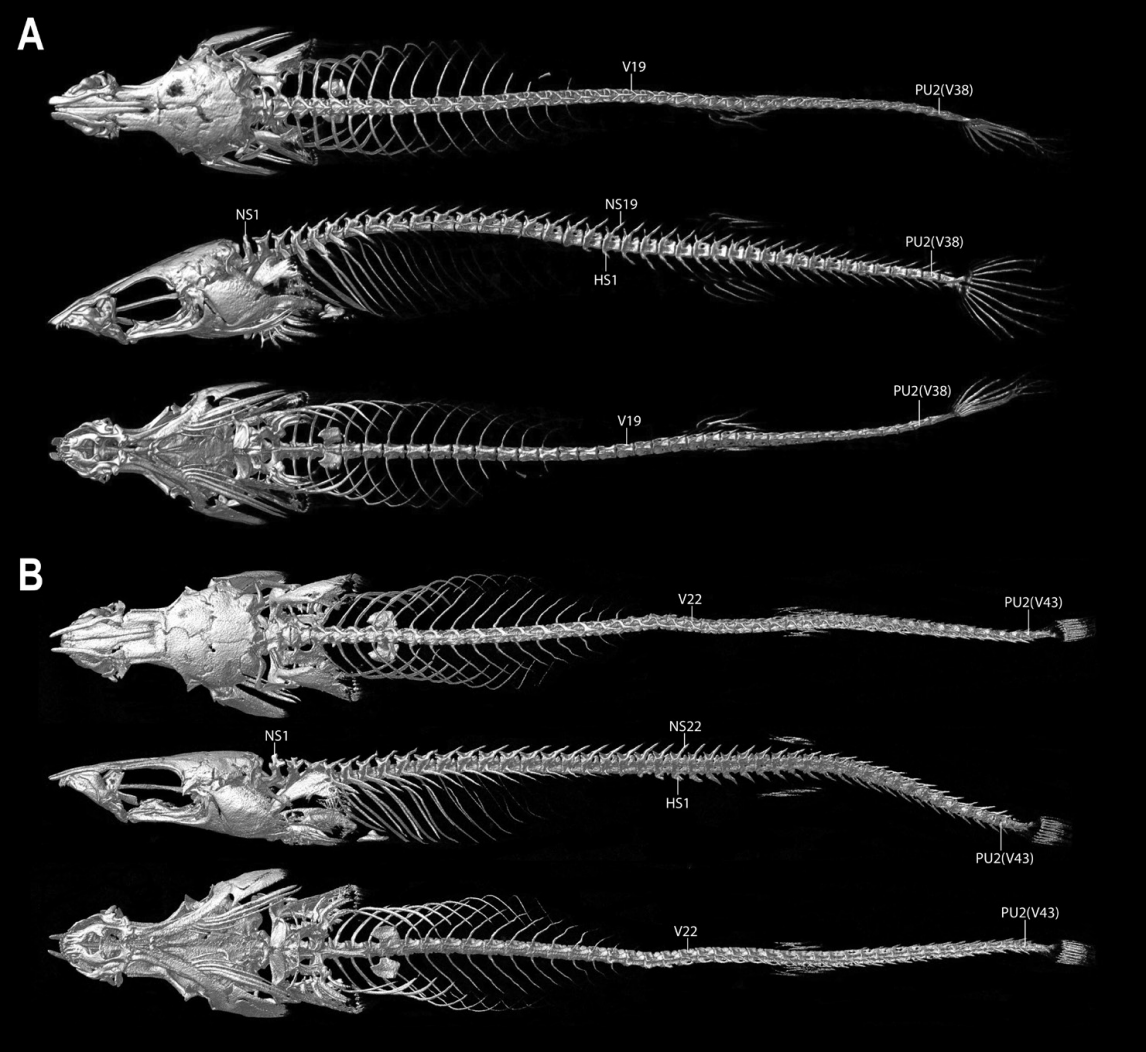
CT scanned skeleton of *Barryichthys* in dorsal, lateral and ventral view A*B.hutchinsi*, AMS I.49000-001, 14.0 mm SLB*B.algicola*, WAM P.27127-016, holotype, female, 16.9 mm SL. Abbreviations: HS, hemal spine; NS, neural spine; PU2, preural centrum 2; V, vertebra.
